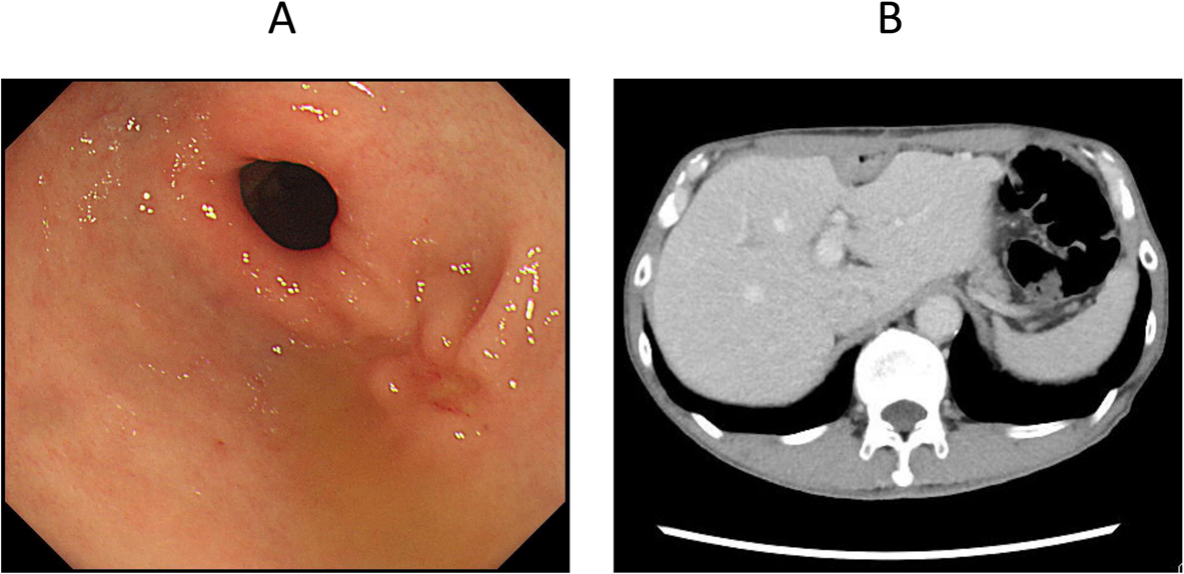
a EGD after chemotherapy. The tumor is significantly reduced and scarred. b CT after chemotherapy. The tumor is reduced and could not be clearly identified
